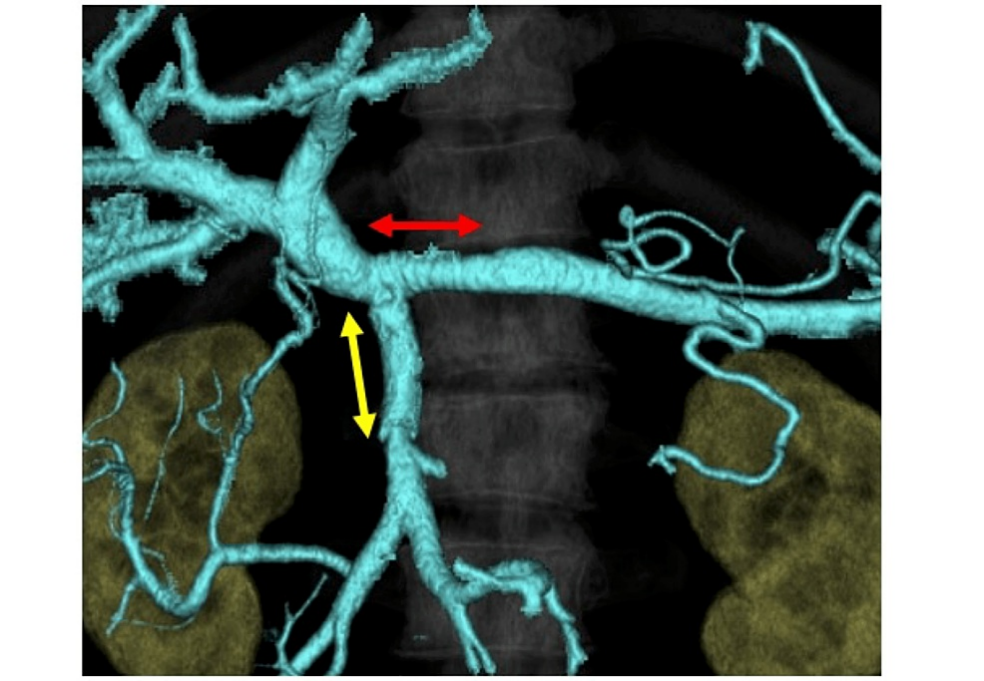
Three-dimensional CT findings after surgery (two months later) Red and yellow arrows indicate anastomosis of the PV-SV and PV-SMV, respectively.
